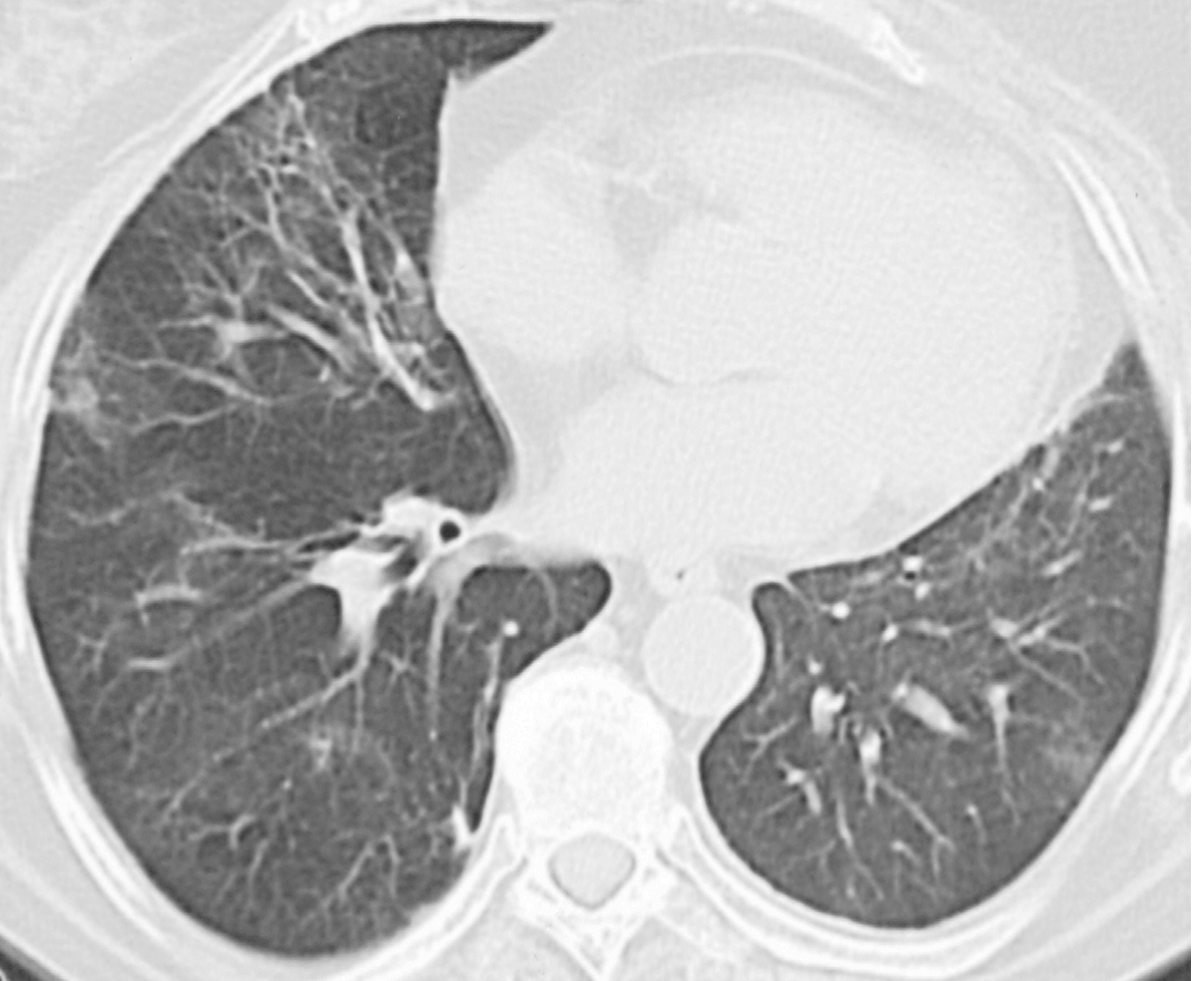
CT shows an obvious improvement of the patchy infiltrates in the right lung after reintroduction of steroids.
